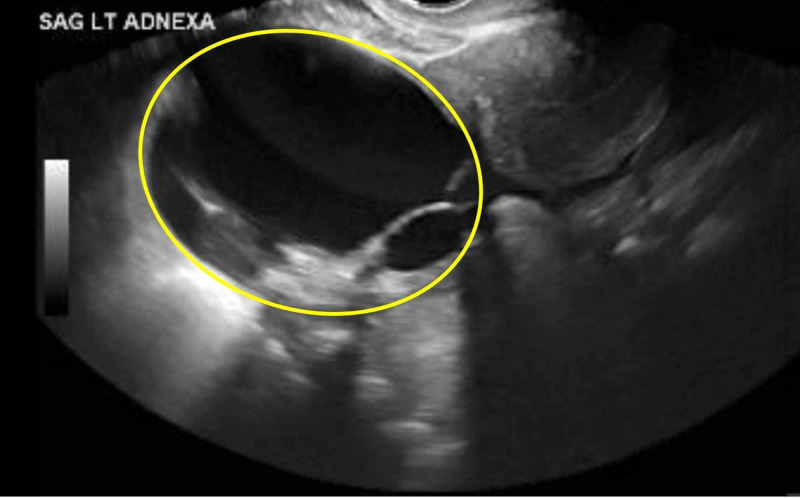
Transvaginal ultrasound showing a stable 8.6-cm multilocular left adnexal cyst with solid component (yellow circle). Color score = 1. O-RADS score = 4. Intermediate risk of malignancy (10 to <50%). O-RADS, Ovarian-Adnexal Imaging-Reporting and Data System
